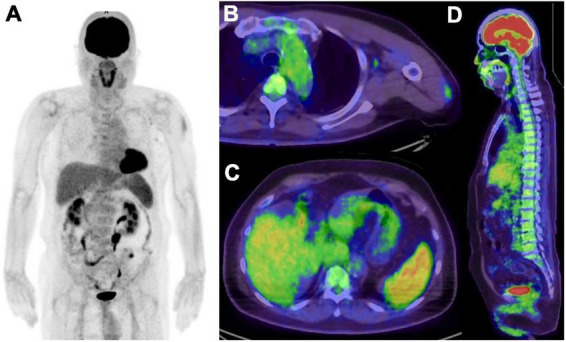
Five days after COVID-19 vaccination in patient suspected recurrence of lung cancer. (A) MIP image of FDG PET, (B,C) axial image of PET/CT, (D) sagittal image of PET/CT. Increased FDG uptake were seen in left deltoid muscle, left axial lymph node, spleen and bone marrow, and all of them related to the vaccination.
